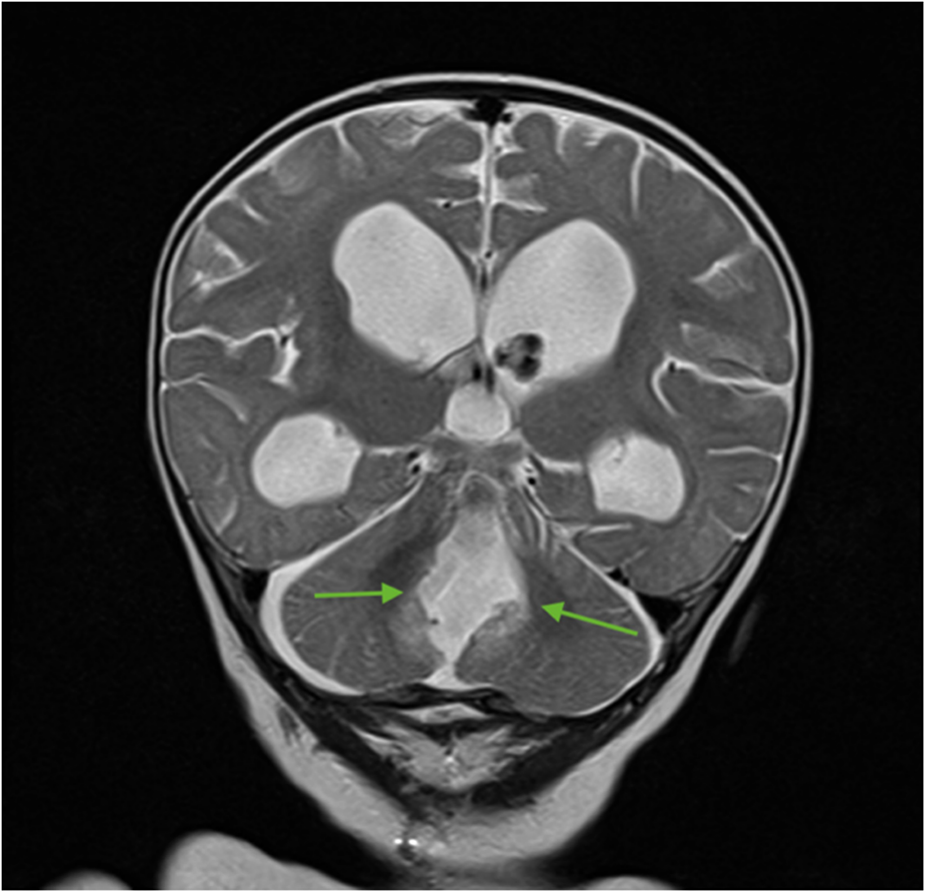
Brain MRI (coronal T2 + C). Postoperative changes, pathologic contrast accumulation in the walls of the resection cavity, and residual tumor in the vermis (green arrows).
